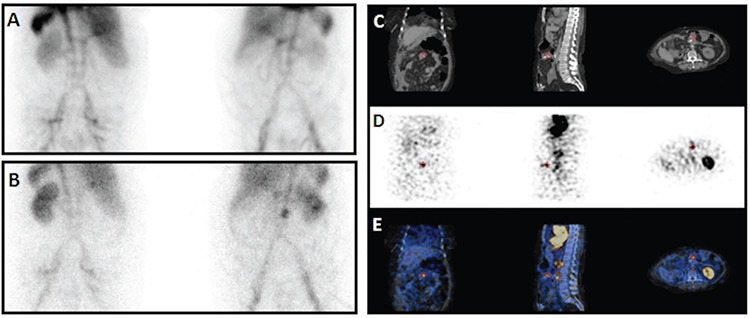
A 73-year-old female with a history of colon cancer, had no signs of bleeding on the 1^st^ day of scintigraphic imaging [(A) posterior and anterior planar images]. At the 24^th^ hour, a focal activity accumulation in the upper quadrant of the abdomen was revealed [(B) posterior and anterior planar images]. SPECT/CT images showed that bleeding focus was at the operating site of colon cancer [(C) CT, (D) SPECT, (E) fused SPECT/CT images in coronal, sagittal, and axial planes, respectively] SPECT/CT: Single photon emission computed tomography/computed tomography
